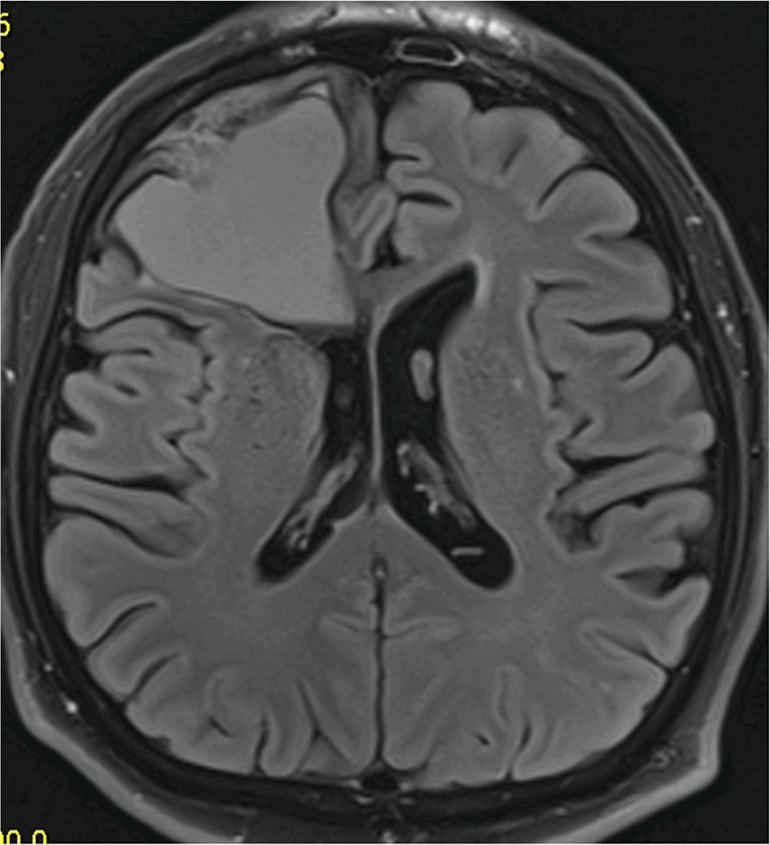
Cyst formation in the right frontal lobe (non-contrast scan). There are clear signs of mass effect, high protein cyst content, and fluid–fluid level (FLAIR transversal scan)
